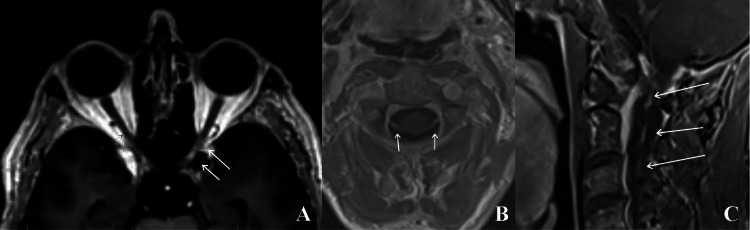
Contrast-enhanced MRI of the brain and cervical spine nerve roots. (A) Axial T1-weighted MRI with contrast. Solid white arrows show abnormal signal changes in the left optic nerve, indicating optic neuritis. This finding is interesting considering that the patient did not demonstrate associated symptoms such as monocular left-eye vision loss or pain with eye movement.
